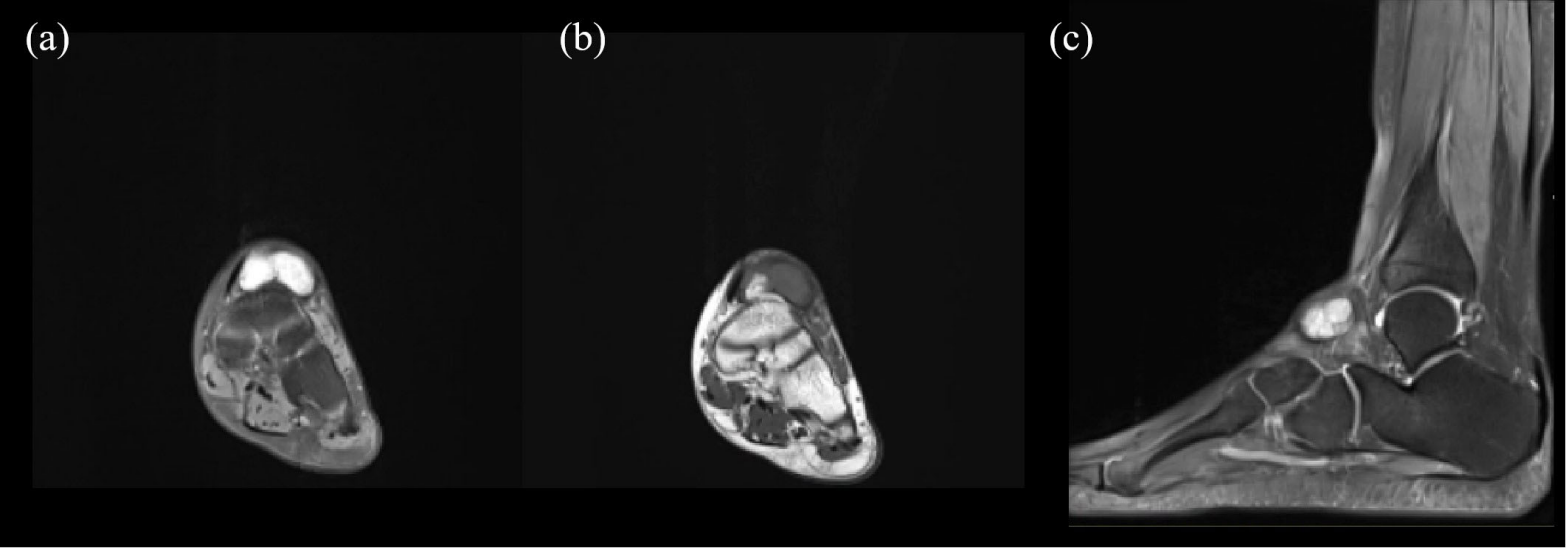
MRI of the ankle reveals a subcutaneous lesion in the left ankle. (a, b) A long T1 and long T2 signal is observed in the subcutaneous tissue of the dorsal foot. (c) Fat-suppressed sequence showed well-defined boundaries with internal septations, displayed from a lateral perspective.
